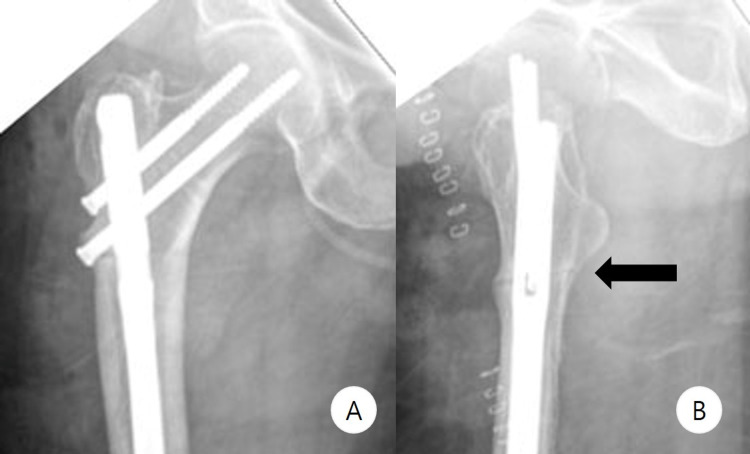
Postoperative imaging - case 2 Postoperative anteroposterior (A) and lateral (B) radiographs show complete fracture line (black arrow) after the reconstruction nail fixation
